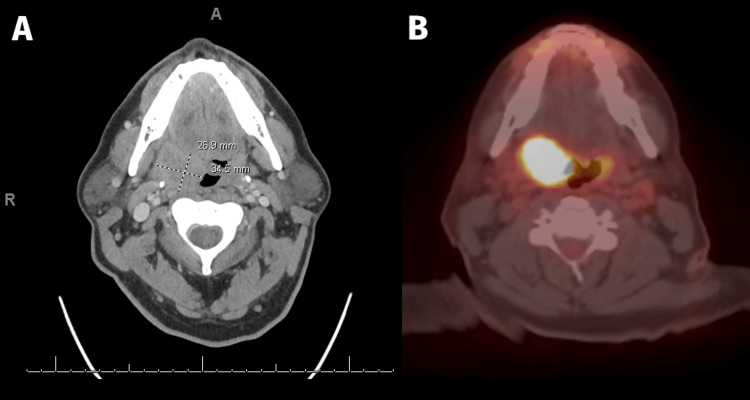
Diagnostic imaging of the primary site. Diagnostic CT (A) and PET/CT (B) imaging showing an FDG-avid 27x35x42 mm mass in the right palatine tonsil.
